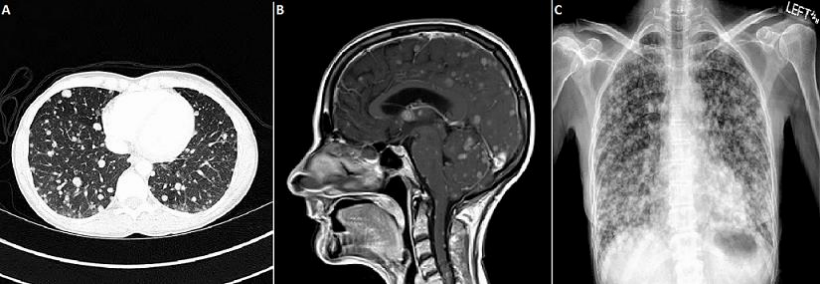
Extensive metastases from large-cell neuroendocrine carcinoma of the cervix: A) chest CT with iodinated contrast; B) sagittal post-gadolinium T1-weighted MRI of the brain and cervical cord; C) chest X-ray
